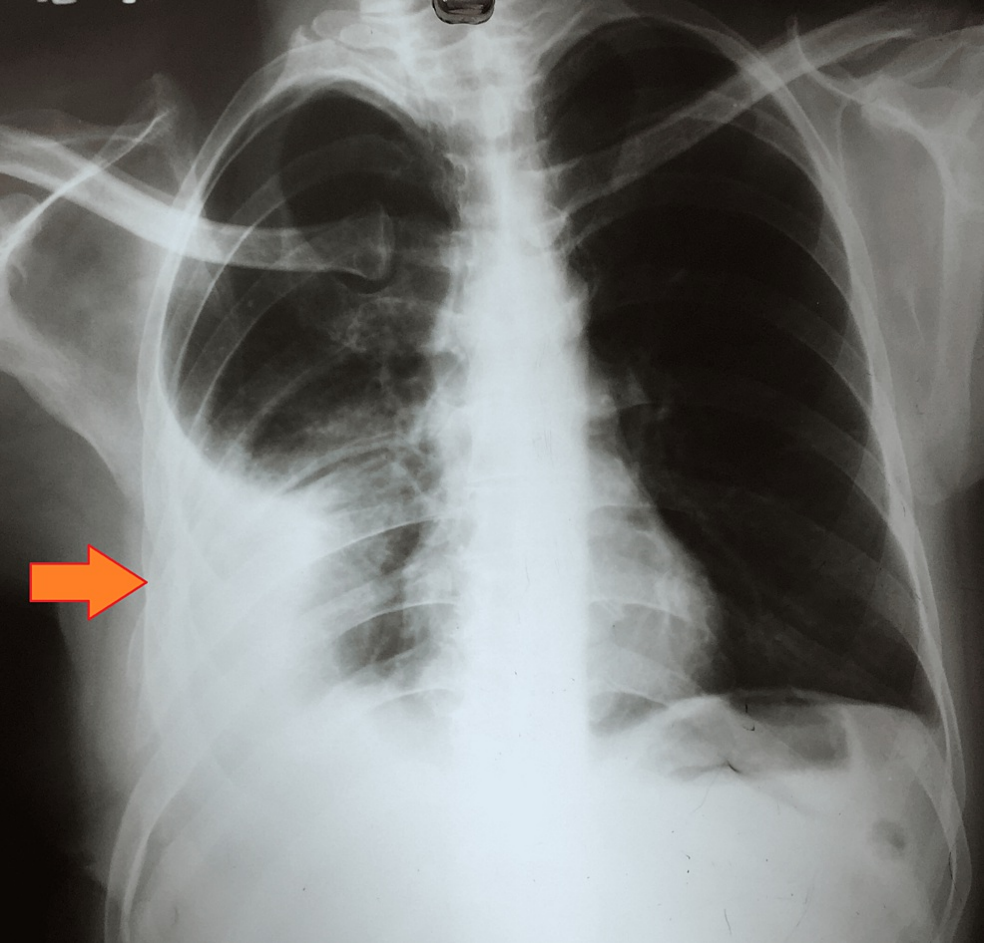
Chest radiograph (P-A) view at the completion of six months P-A: postero-anterior
